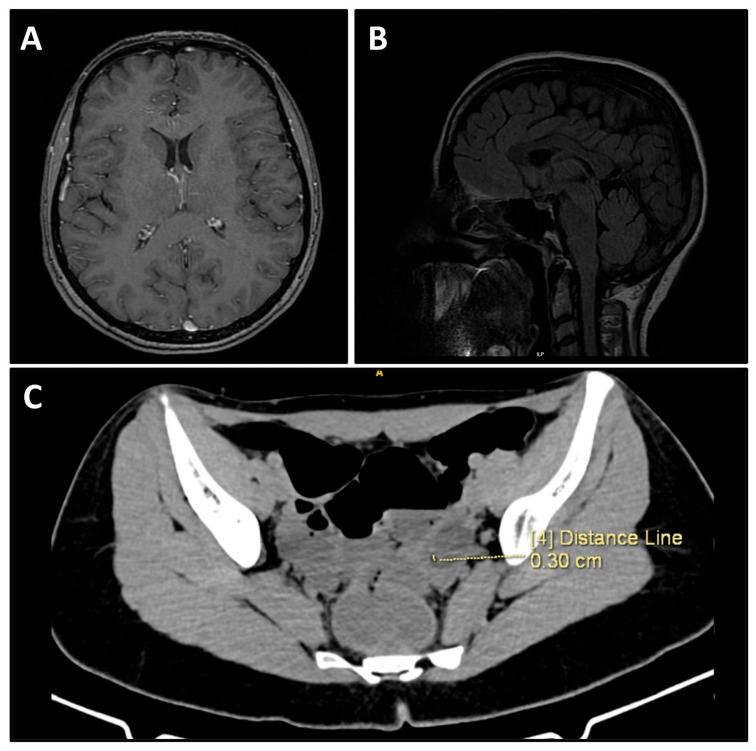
Imaging diagnostics. (A) Normal axial MRI of the brain in T1. (B) Normal sagittal MRI of the brain in T2 FLAIR. (C) CT scan of the lesser pelvis demonstrating a 27 × 19 mm cystic lesion in the left ovary.
